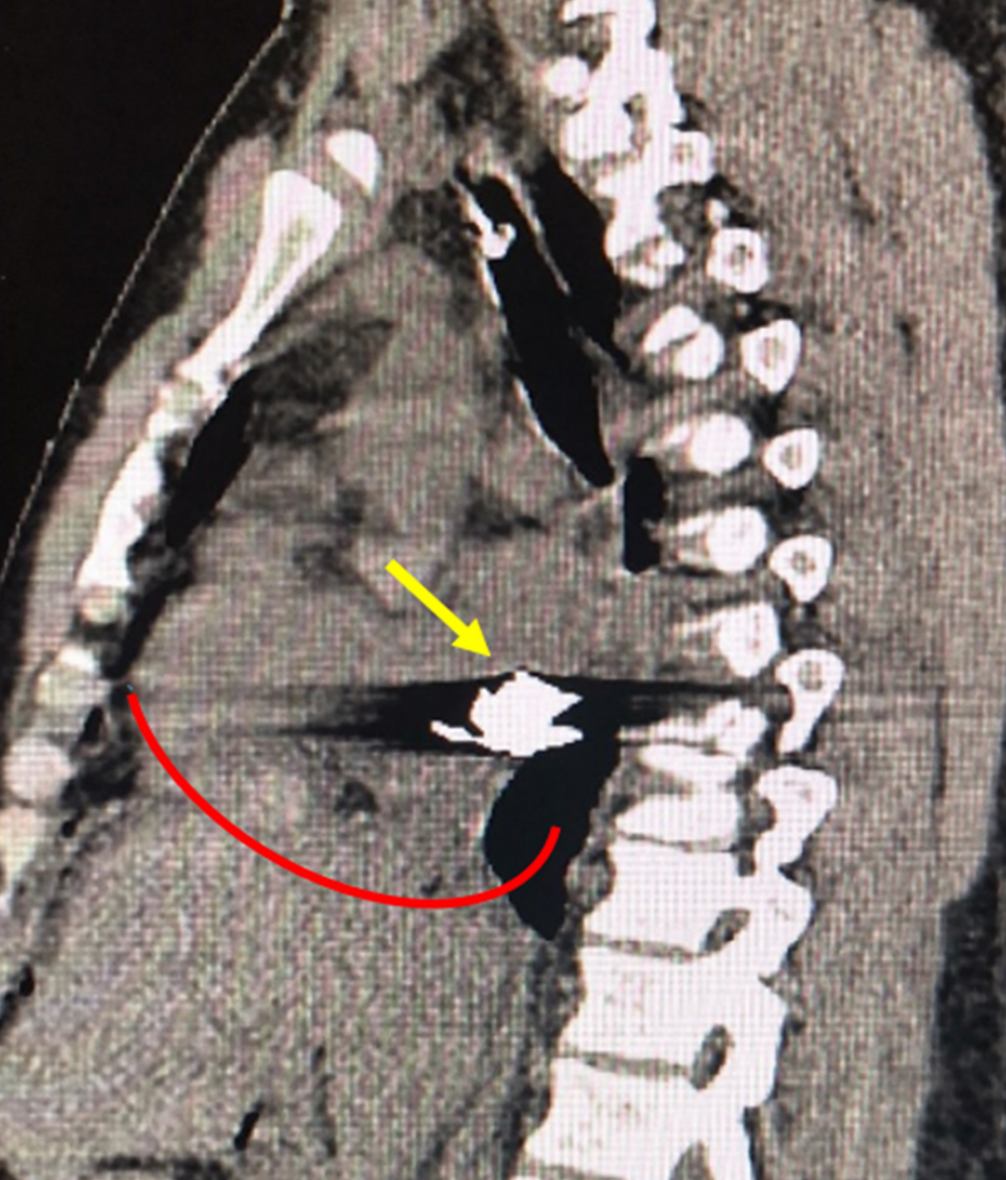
Post-Surgical Confirmatory Non-Contrast Sagittal Computed Tomography of the Thorax A post-surgical non-contrast sagittal computed tomography of the thorax confirms the anatomic and spatial location of a bullet embolus (yellow arrow) that migrated from the patient's inferior vena cava into the right atrium. The inferior heart border is delineated by the curved red line.
